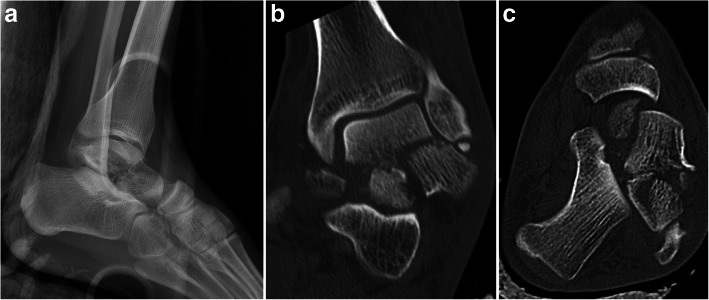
Concomitant head, body & neck fracture of the talus associated with talonavicular subluxation in a young man. Radiograph (a), CT sections (b & c)
